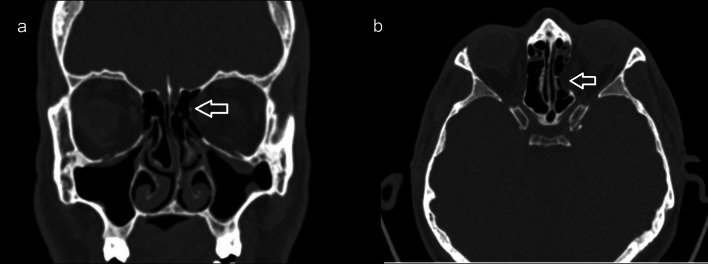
45-Year-old male patient with left (arrow) lamina papyracea dehiscence on coronal (a) and axial (b) paranasal CT image
